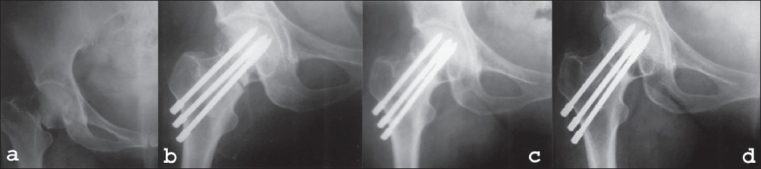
X-ray anteroposterior view (a) showing femoral neck fracture in a 49-year-old female patient (b) and immediate postoperative X-ray of the same patient, (c) X-ray anteroposterior view of the same patient at follow-up at four months (d) and at two years shows fracture consolidation and absence of AVN
